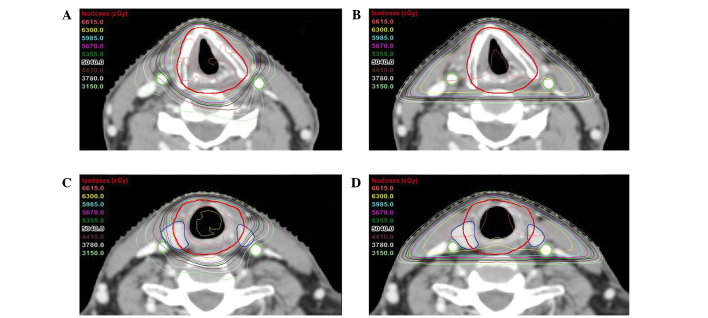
Comparison of the isodose distributions of the two RT techniques in a representative case. (A) VMAT and (B) conventional RT axial plan CT images at the mid-PTV (red) level showing the carotid artery (green). (C) VMAT and (D) conventional RT axial plan CT images at the lower PTV level showing the thyroid gland (blue). RT, radiotherapy; VMAT, volumetric modulated arc therapy; CT, computed tomography; PTV, planning target volume.
